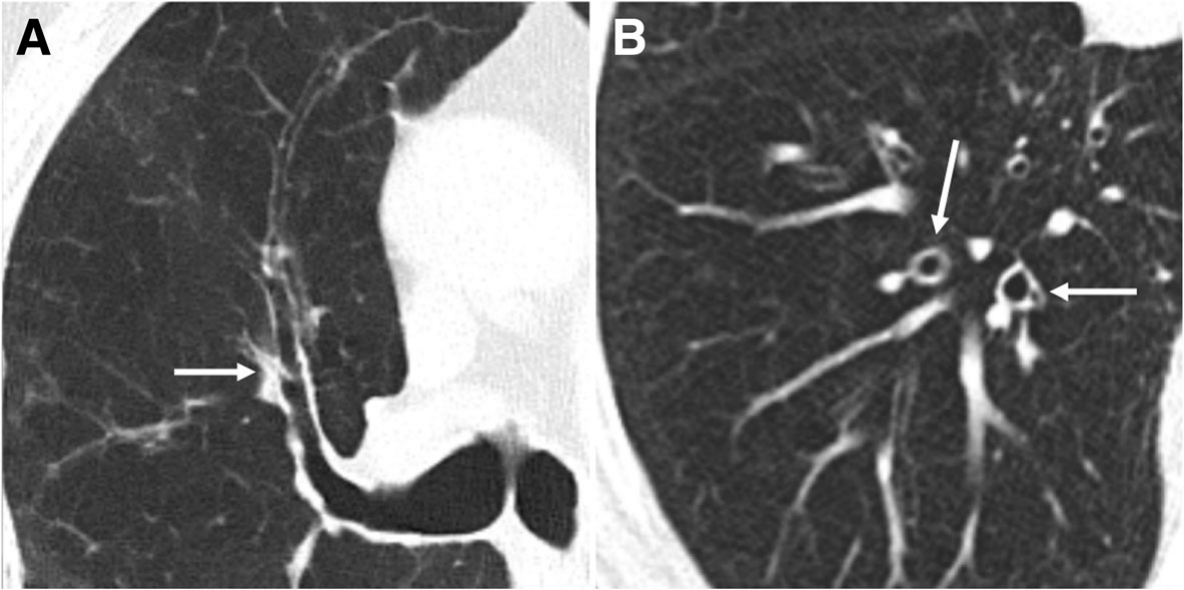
Bronchial wall thickening in a 79-year-old heavy smoker man. a and b Axial images from a chest CT show thickening of the bronchial walls (arrows). Bronchial wall thickening is an important predictor factor of FEV1 and of the risk of acute exacerbation
